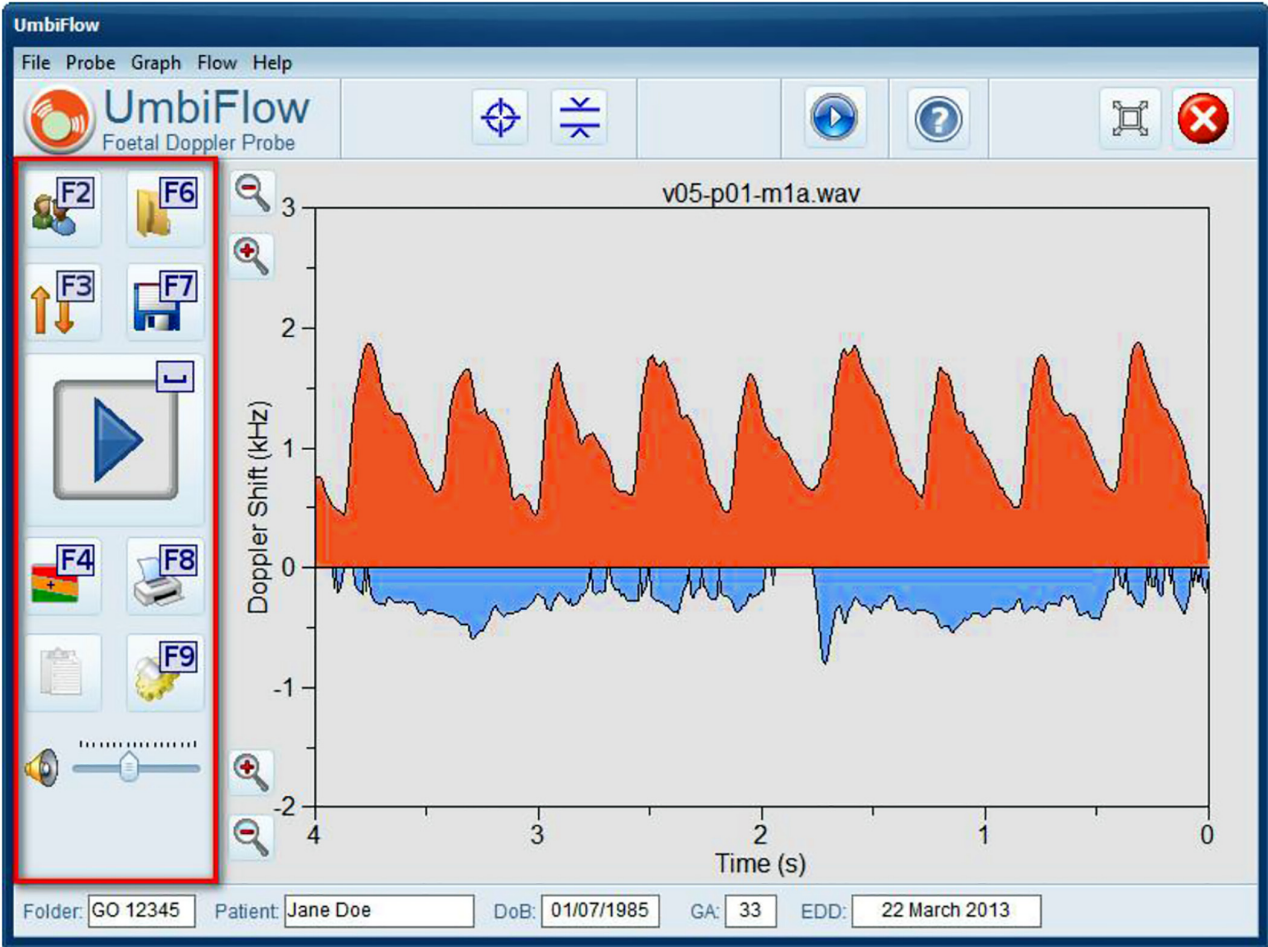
User interface of UmbiFlow™ Doppler software.
